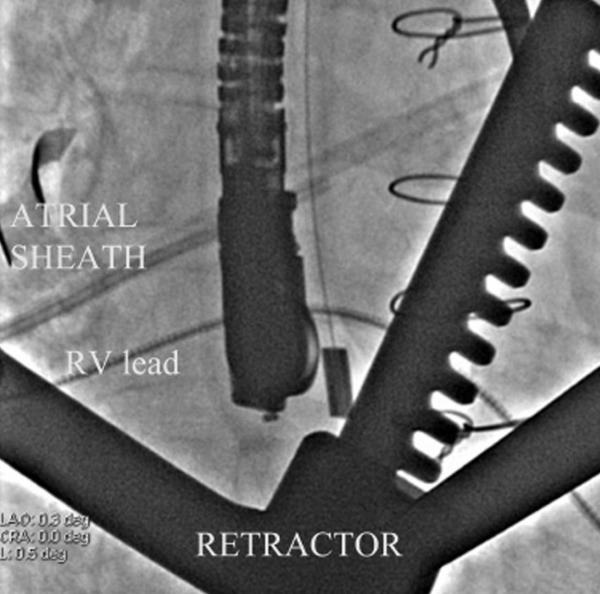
Figure 1 shows frontal plane fluoroscopy with retractors through the right anterolateral thoracotomy, transesophageal echocardiographic probe (TEE) guides placement of atrial and ventricular (RV) screw-in leads.
